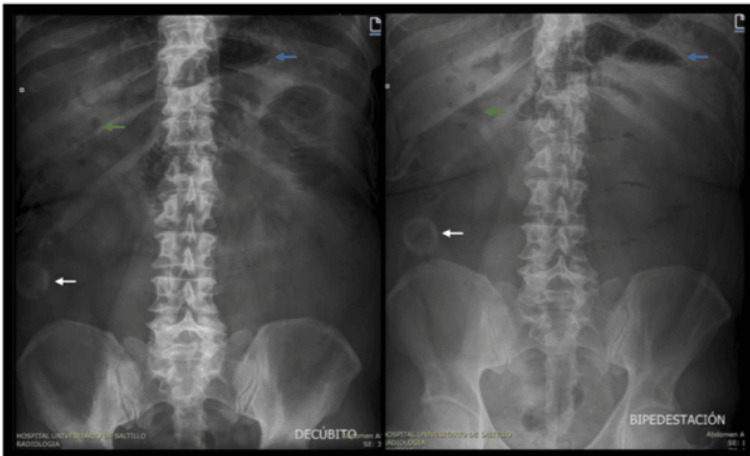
Plain abdominal radiograph in two positions. Green arrow: pneumobilia, blue arrow: dilation of intestinal loops, air-fluid levels, white arrow: gallstone.
